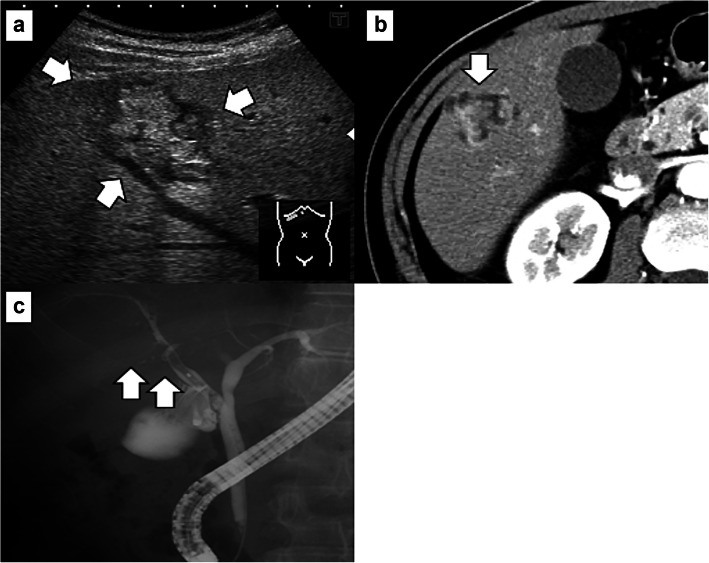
Preoperative imaging findings. a Abdominal ultrasonography revealed a hyperechoic tumor (core size of 20 mm) in the intrahepatic area and peripheral to the hypoechoic area (white arrow). The hyperechoic area is even more intense at the posterior aspect of the tumor. b Dynamic computed tomography revealed an unevenly shaped and enhanced tumor (white arrow) in S5 of the liver during the arterial phase. c Endoscopic retrograde cholangiography revealed a filling defect (white arrow) from the origin of the right area to the periphery and severe dilation of the S5 branch of the bile duct
